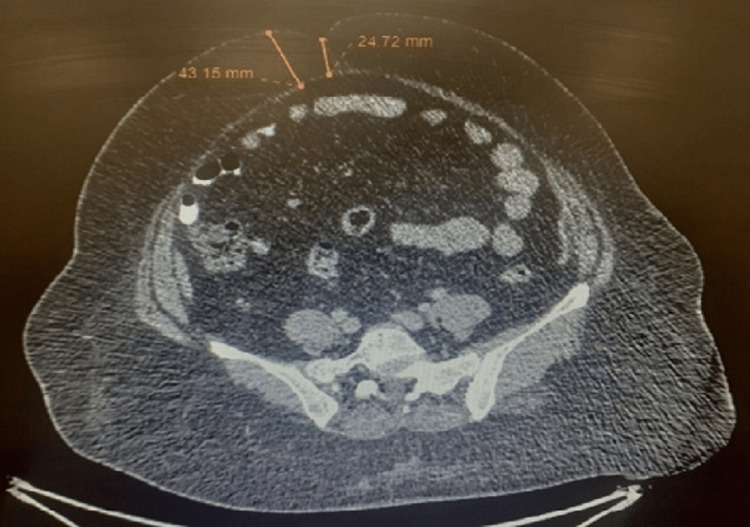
Cross-sectional cut of the preoperative CT scan at the level of the umbilicus showing a 4.15 cm depth of subcutaneous fat layer in the anterior abdominal wall
